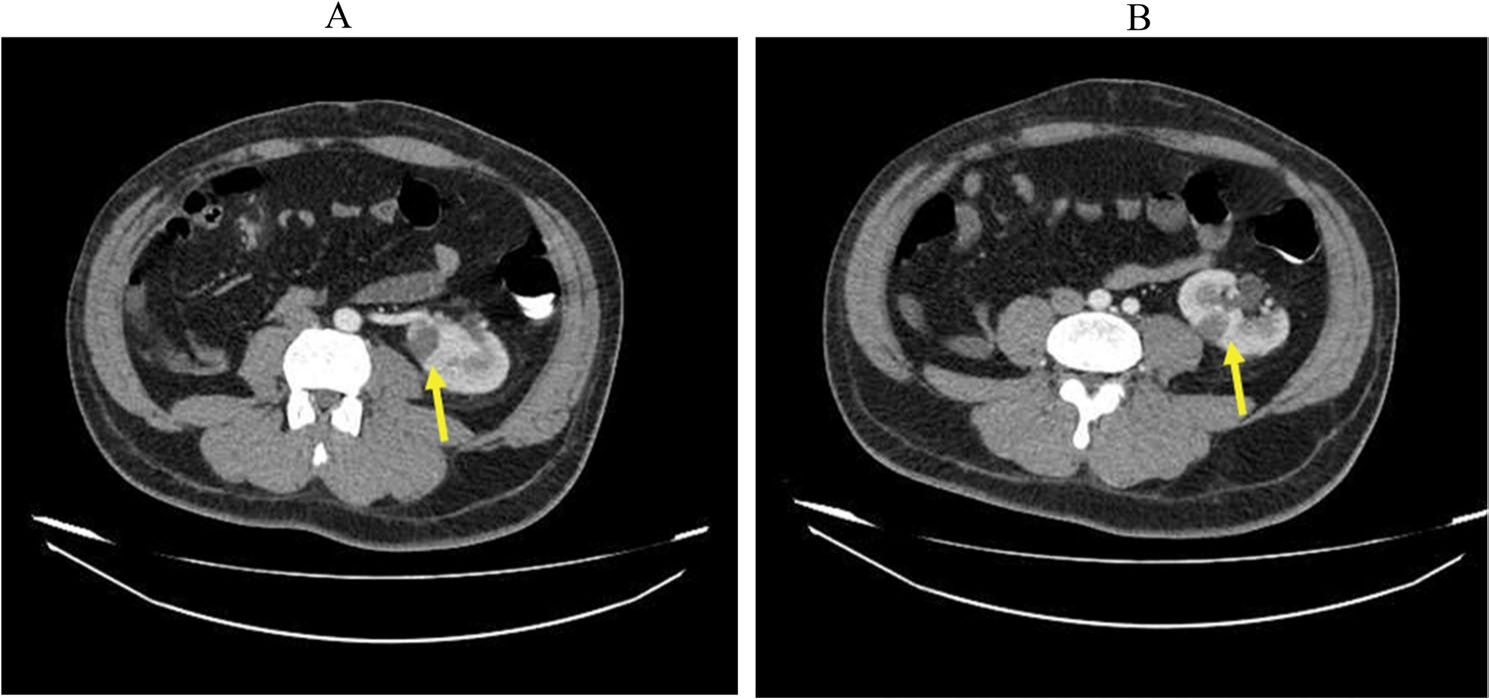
Axial Abdominopelvic CT with intravenous contrast. A Wedge-shaped hypoenhancement in the left kidney suggesting renal infarction. B Another slice from the same abdominal CT scan, demonstrating the same lesions. (Arrows indicate areas of infarction)
